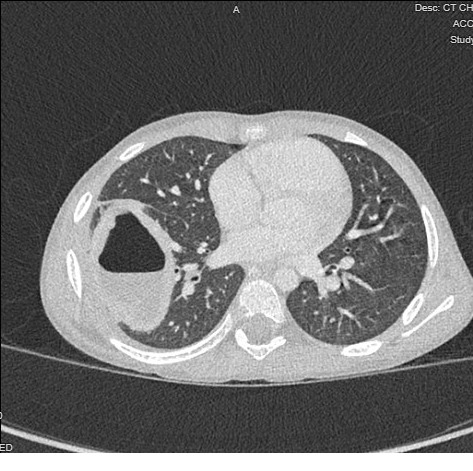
Repeat chest CT during the patient’s third hospital encounter.
